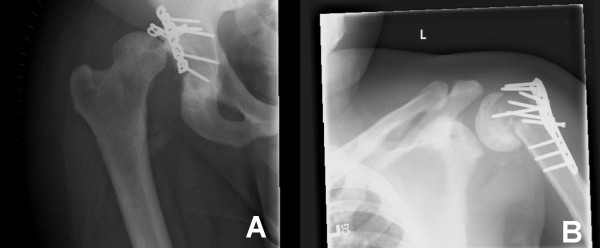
Radiographs of failed hardware after non-compliance with weight bearing, hip position, and ongoing substance abuse.
